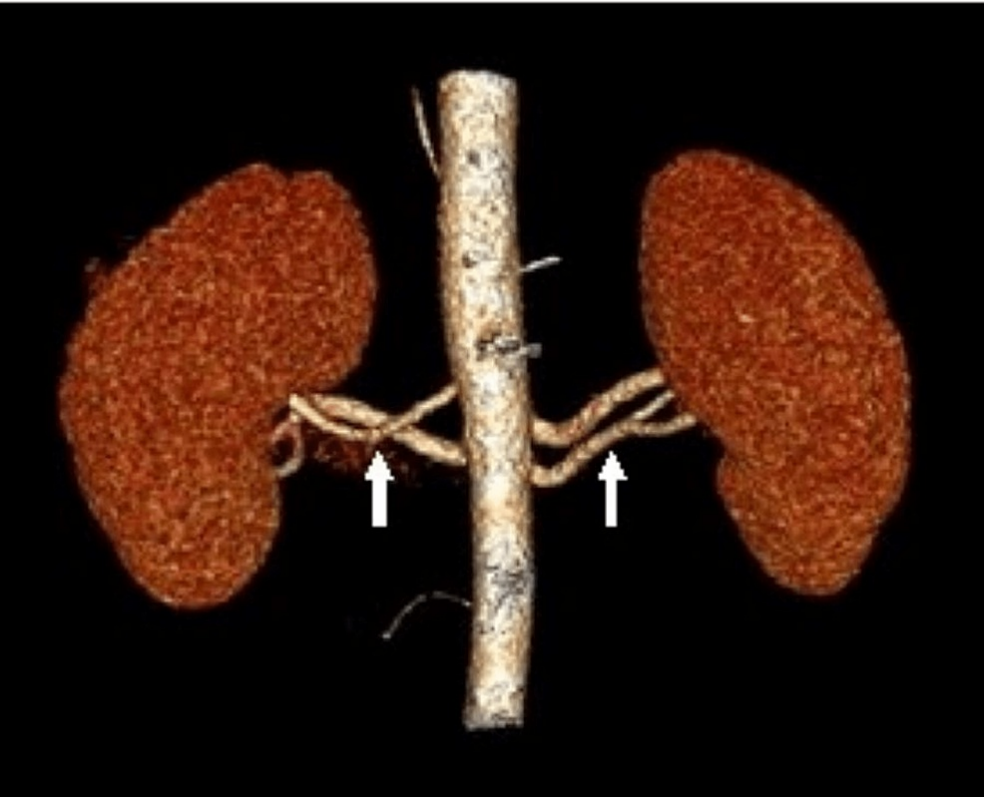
Multiplanar reconstruction image of CT renal angiography revealed bilateral accessory renal arteries separately originating from the aorta.
